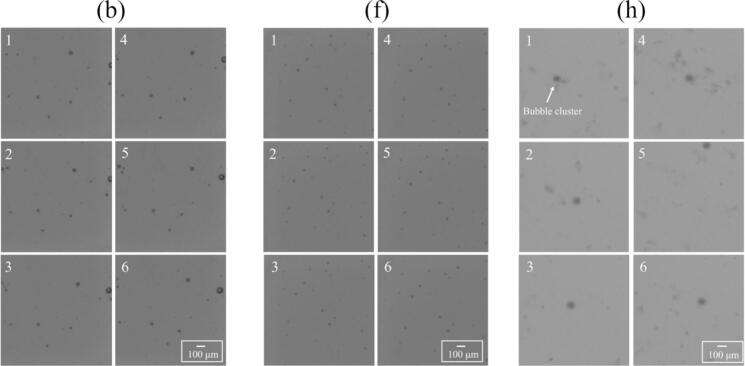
Direct observation of bubbles taken by a highspeed camera with the ultrasonic power of (b) 33 W, (f) 84 W, and (h) 136 W. The time interval of each picture was 0.06 sec. (Supplemental Video2).
